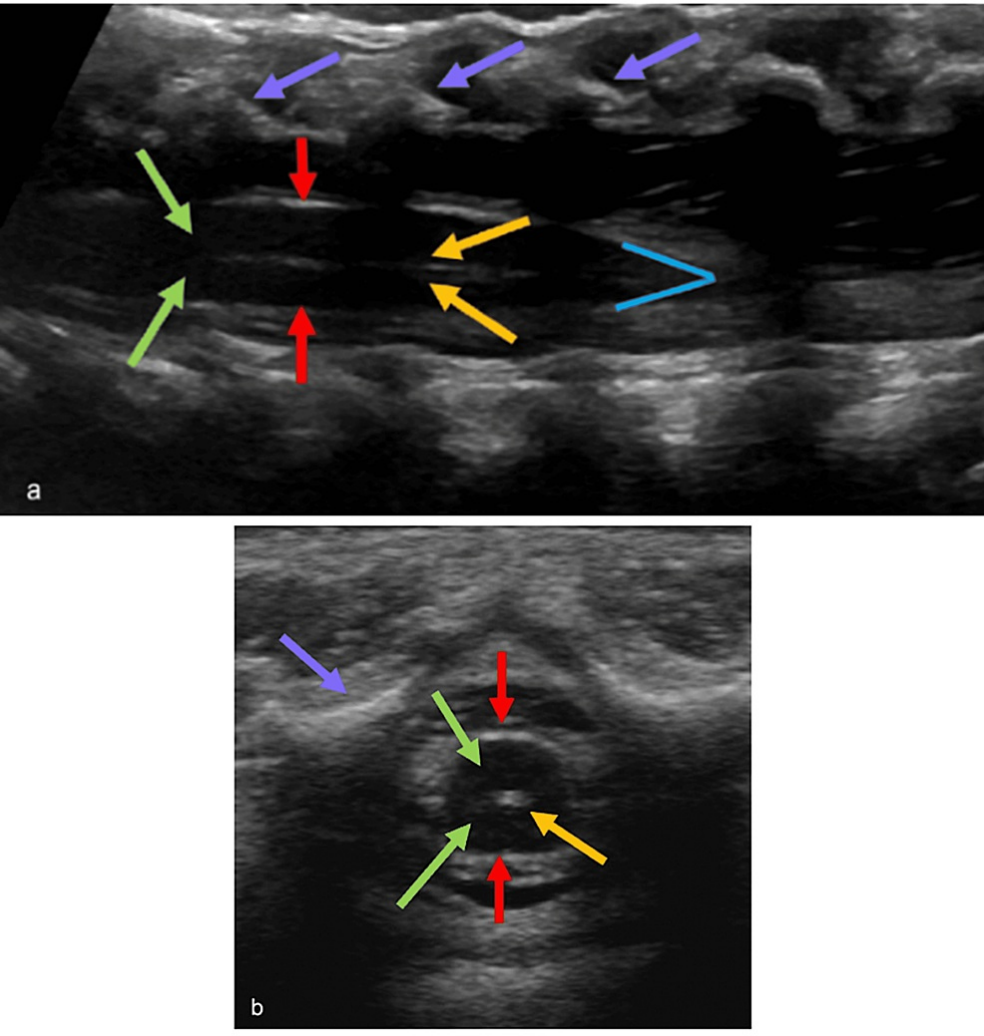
Normal cord anatomy on ultrasound Longitudinal (a) and transverse (b) ultrasound images demonstrating normal cord anatomy. The vertebrae appear as echogenic structures anterior to the cord (purple arrows). The spinal cord is a tubular hypoechoic structure with hyperechoic walls (red arrows). The central echo complex is demonstrated by an echogenic structure located centrally within the spinal cord (yellow arrows). Hypoechoic subarachnoid space surrounds the cord (green arrows). The conus medullaris appears as a V-shaped tapering of hyperechoic walls at the end of the cord (blue V) Radiological images obtained by the authors
